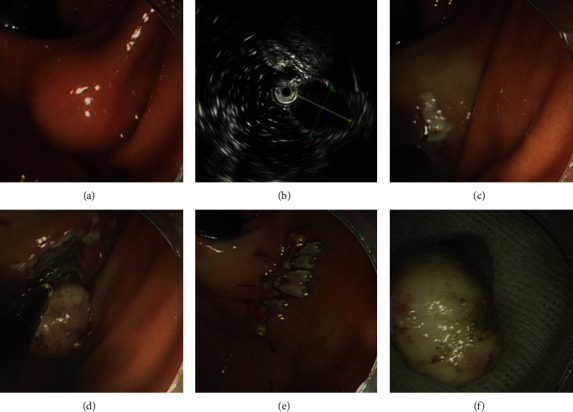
Endoscopic submucosal dissection treatment for a gastric GIST: (a) gastric GIST identified by endoscopy; (b) the same gastric GIST observed by endoscopic ultrasound; (c) marking outside the tumor; (d) dissection of the tumor; (e) close the wound; (f) the resected tumor. GIST: gastrointestinal stromal tumor.
